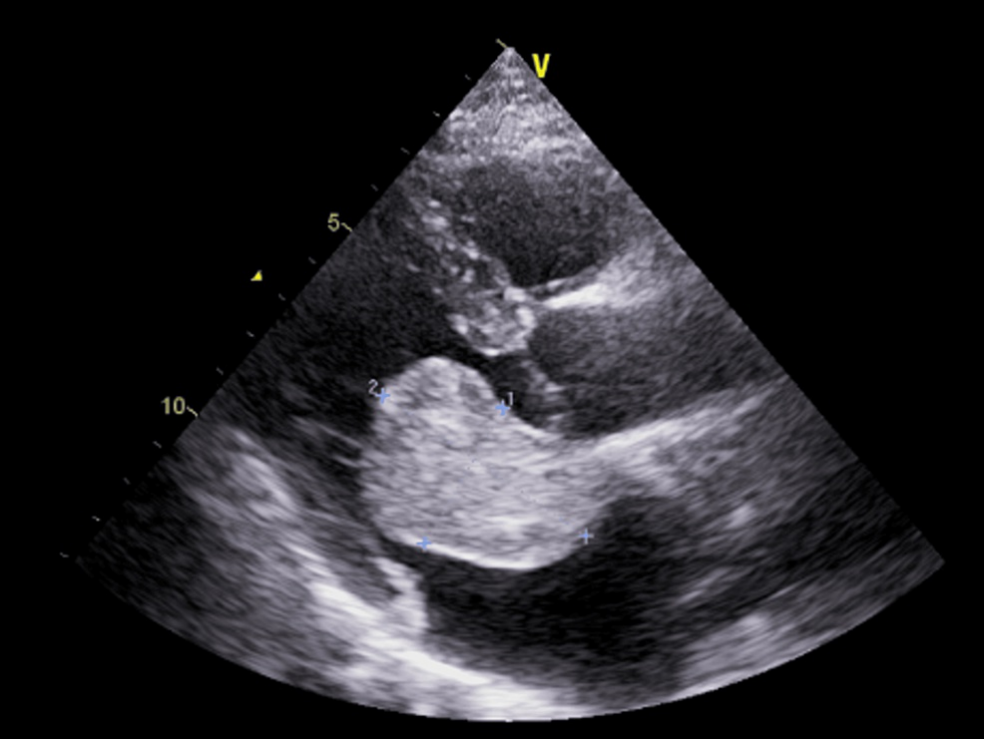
Echocardiography showing a 3.7 x 5.1 cm tumor in the left atrium protruding in the left ventricle
